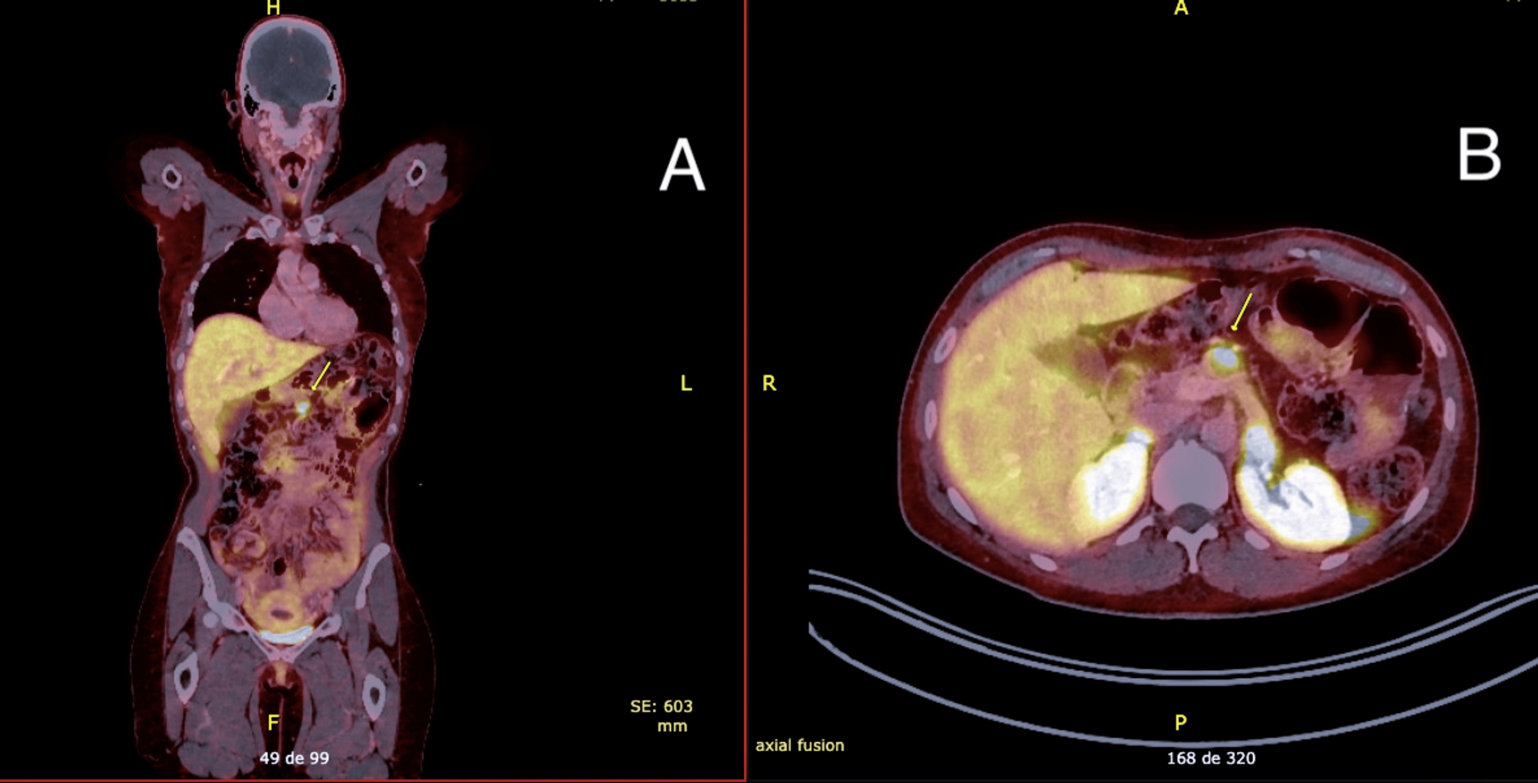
PET-CT with octreotide: (A) coronal plane and (B) axial plane Arrows: hyperuptake in the pancreas (maximum SUV: 7.5) with no other lesions, consistent with neuroendocrine neoplasia SUV: standardized uptake value
